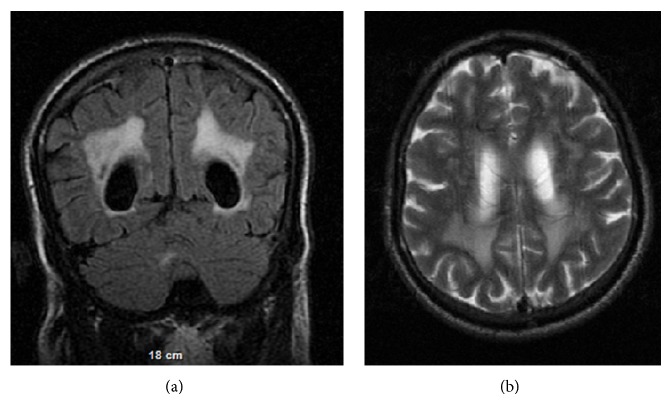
Patient number 19: a 46-year-old man with severity score 18. (a) Coronal FLAIR image shows bilateral dense parietal and occipital hyperintensities, confluent in the posterior periventricular areas and significant enlargement of ventricles. (b) Axial T2W image demonstrates round subcortical bilateral frontal white matter hyperintensities and significant enlargement of ventricles and subarachnoid spaces (severity of brain atrophy: 3 points).
